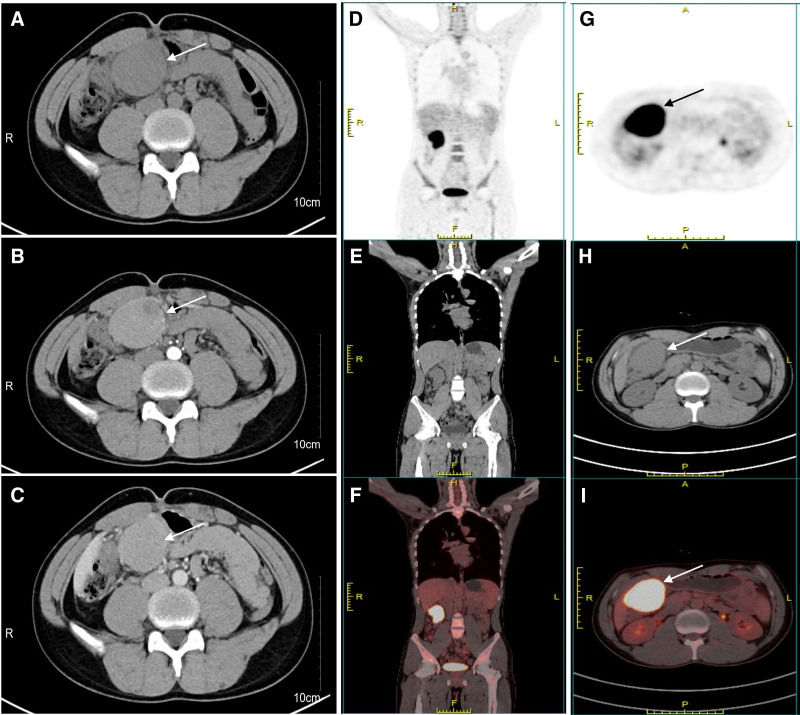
Computed tomography and 18F-FDG PET/CT image of gastric FDCS. (A) Unenhanced axial CT showed well-defined, homogeneously hypoattenuating mass (arrow) with approximately 68 mm × 47 mm × 91 mm in the right mid-abdomen. (B and C) Contrast-enhanced axial CT showed the tumor being heterogenous strengthened, enhanced scan continues to be strengthened. (D–I) focal hypermetabolism of the lesion corresponding to the mass in CT. CT = computed tomography, FDCS = follicular dendritic cell sarcoma, PET = positron emission tomography.
